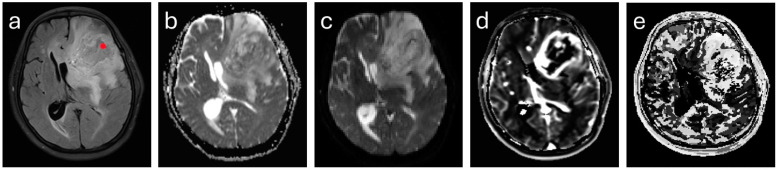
Exemplary maps of one patient with glioblastoma in the left frontal lobe. FLAIR (a), apparent diffusion coefficient (ADC) (b), mean kurtosis (MK) (c), dynamic contrast-enhanced (DCE) MRI parameter Ktrans (d), and time to peak (TTP) (e). The red mark indicates the biopsy ROI.
